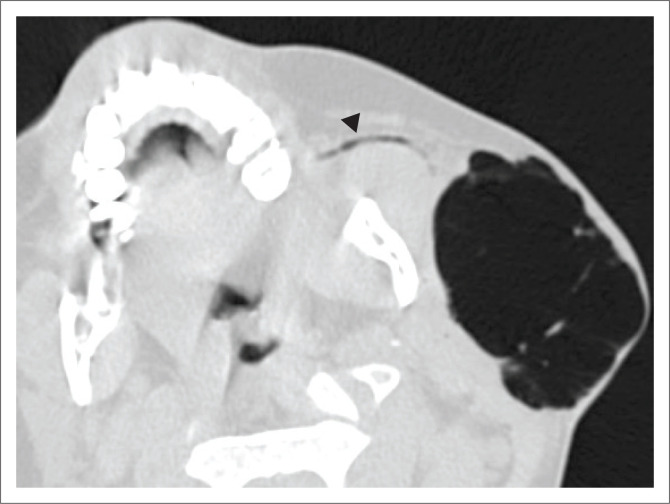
Magnified axial non-contrasted computed tomography demonstrating gas in the mildly distended left Stenson’s (parotid) duct (black arrowhead).
